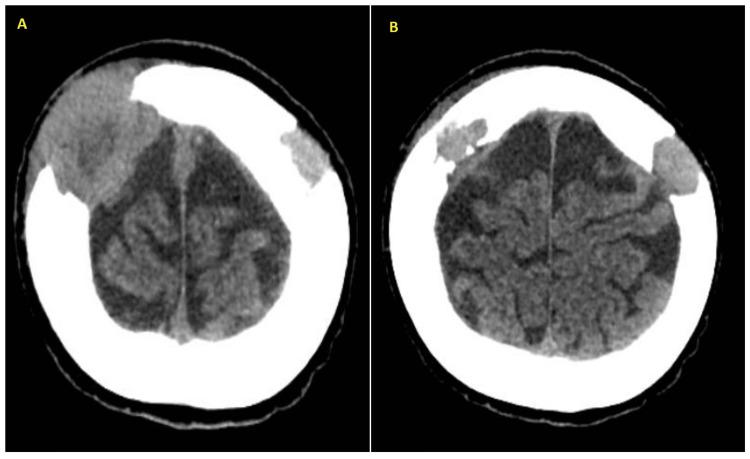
A brain CT scan shows a lytic lesion at the right parietal bone involving the inner and outer tables associated with a soft tissue component with intracranial extension (A) and a lytic lesion at the left parietal bone involving the inner and outer tables associated with a soft tissue component without intracranial extension (B).
